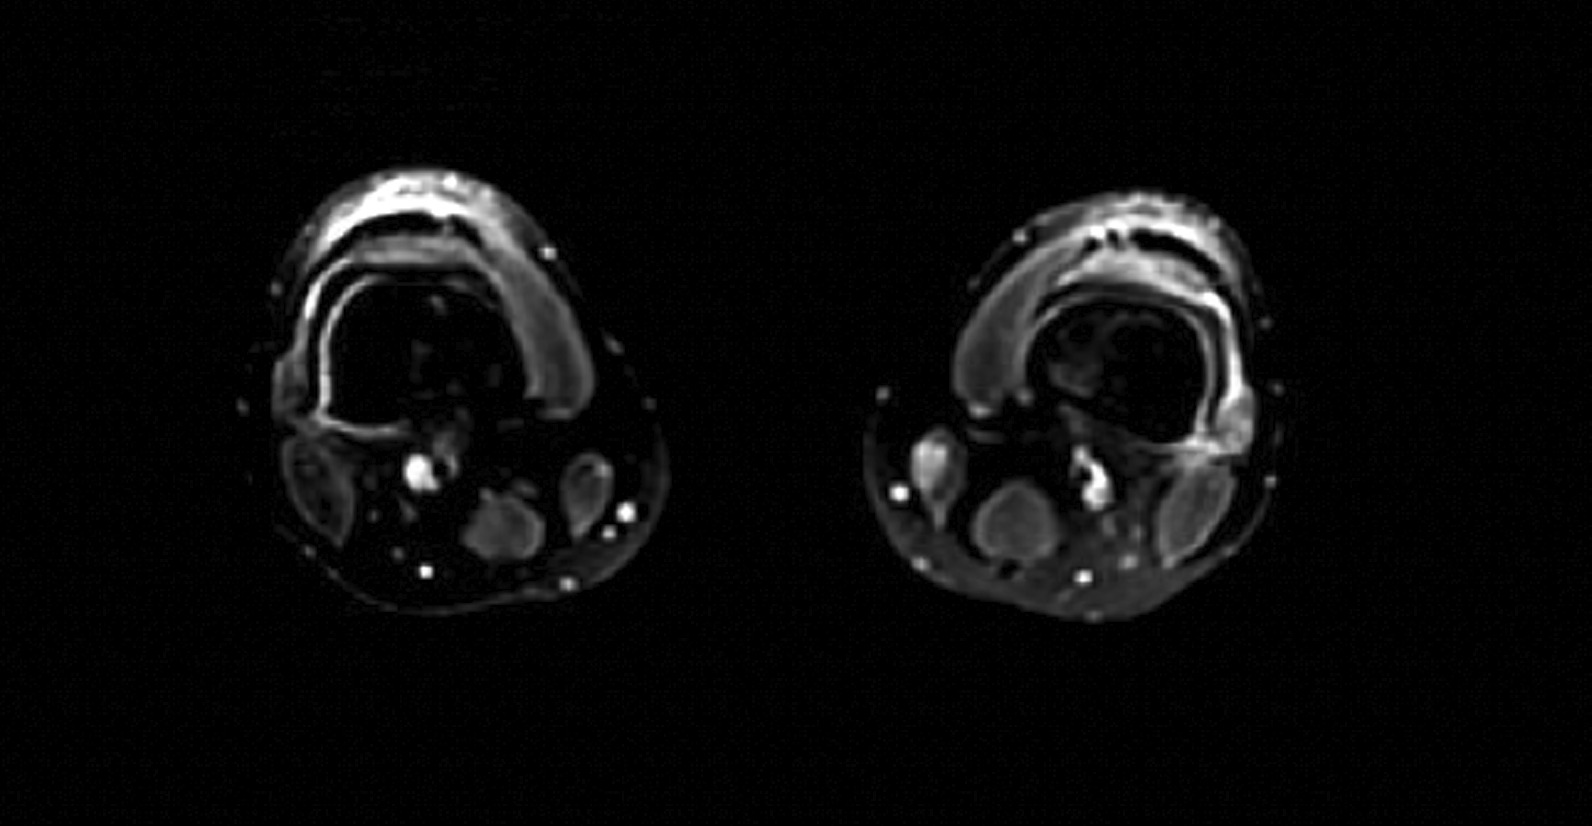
MRI of lower extremities showing nonspecific edema of left distal sartorius muscle
